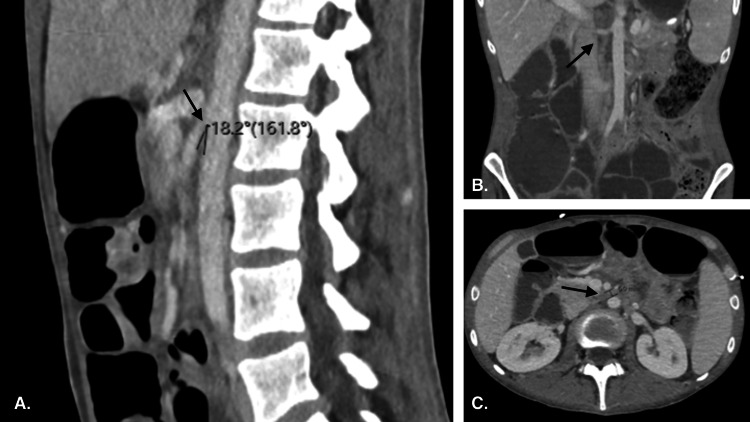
A contrast-enhanced computed tomography (CT) of the thorax, abdomen, and pelvis is shown. The CT revealed a loss of fat pad between the SMA and aorta, resulting in duodenal compression with a shortened SMA-aortic angle (arrows). 1A: sagittal view; 1B: coronal view; 1C: axial view SMA: superior mesenteric artery
